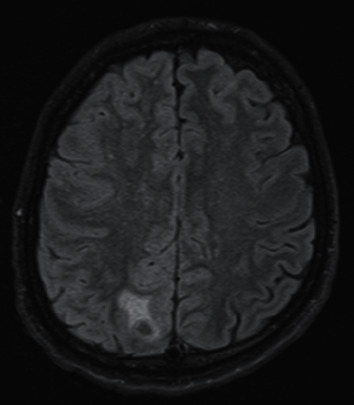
MRI flair image at the same level depicts oedema surrounding a small, nodal lesion.
